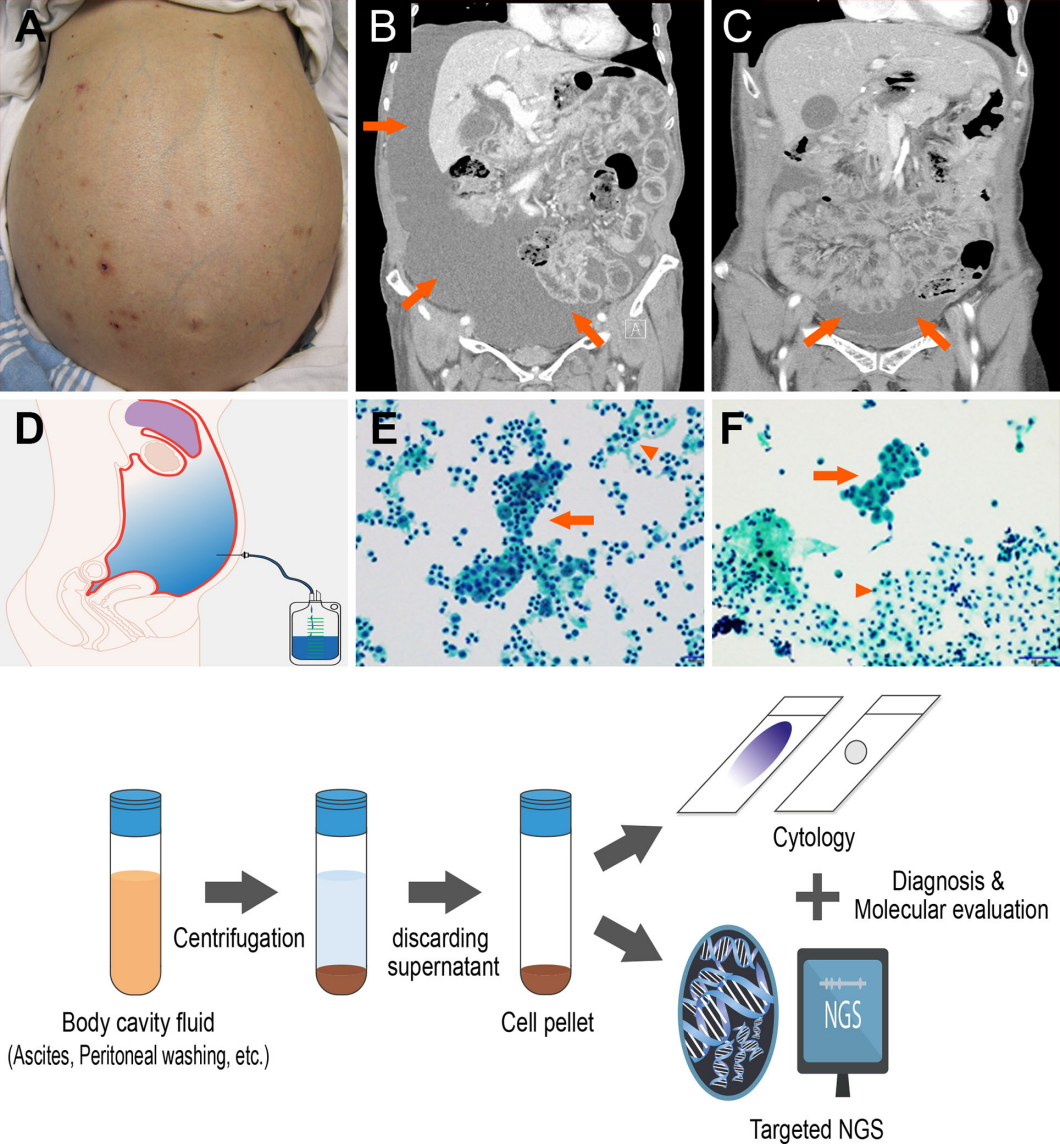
Ascites and peritoneal washing cytology from the gastrointestinal cancer patients (A) Patients with swollen abdomen with massive ascites. Abdominal computed tomography scans showing (B) a large amount of ascites, (C) mild ascites irrigated with peritoneal washing. (D) Cytology samples can be collected from paracentesis. (E) Ascites and (F) peritoneal washing cytology contains tumor cells (arrow) with abundant background mesothelial cells and inflammation cells (arrow head). (Below) Cytology sample processing to diagnosis and targeted sequencing.
